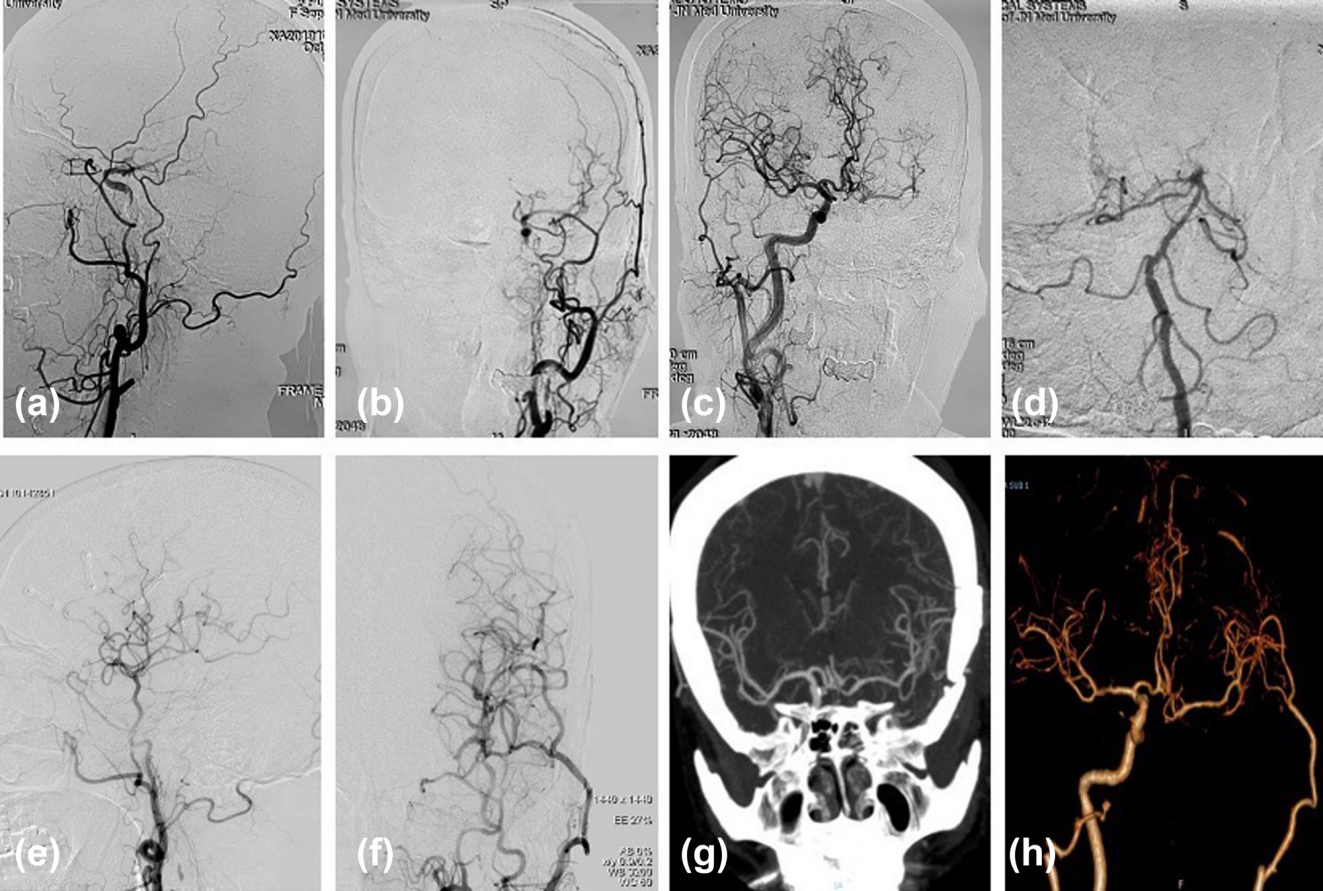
Patient with left ICAO. DSA imaging before (a–d) and after (e–f) STA–MCA double bypass. CTA images (g–h) were obtained 1 year after the bypass surgery.
